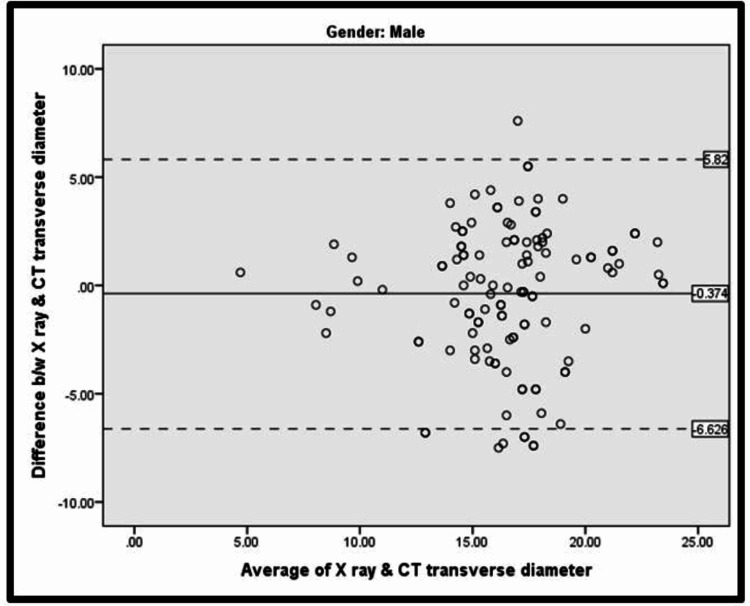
Bland-Altman plot for X-ray vs CT transverse diameter among males The solid line shows bias, and the dotted lines show the limit of agreement.
